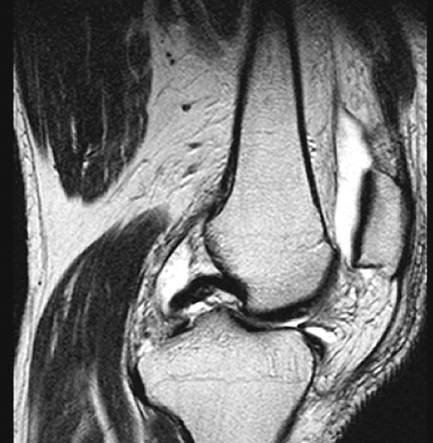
The grade III lesion of the medial tibial plateau in Fig. (2A) was interpreted to be grade II in magnetic resonance image. Grade I irregularity of the medial femoral condyle was not detected in MRI.
